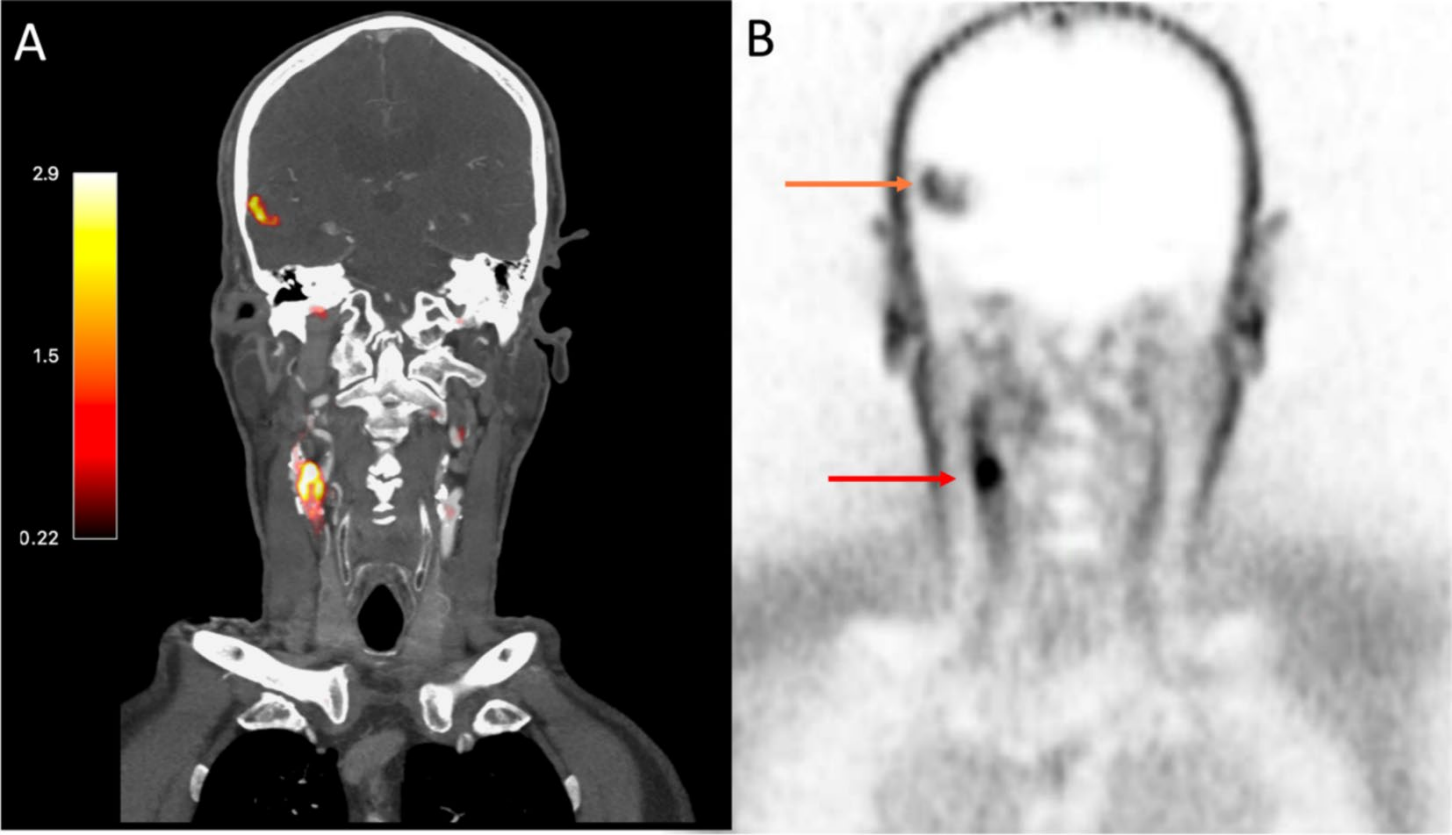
^18^F-GP1 Positron Emission Tomography images of a patient with an acute ischaemic stroke caused by large artery atherosclerosis. Images from an 81-year old gentleman presenting with headache and confusion. Panel (A) shows fused computed tomography and positron emission tomography images with PET uptake seen as red/orange. Corresponding PET only images in Panel (B). Both panels show^18^F-GP1 uptake in right carotid artery (Panel (A)—red/orange colour and Panel (B)—red arrow) indicating acute plaque rupture with thrombus embolization causing right middle cerebral artery infarct (Panel (A)—red/orange colour, Panel (B)—orange arrow).
